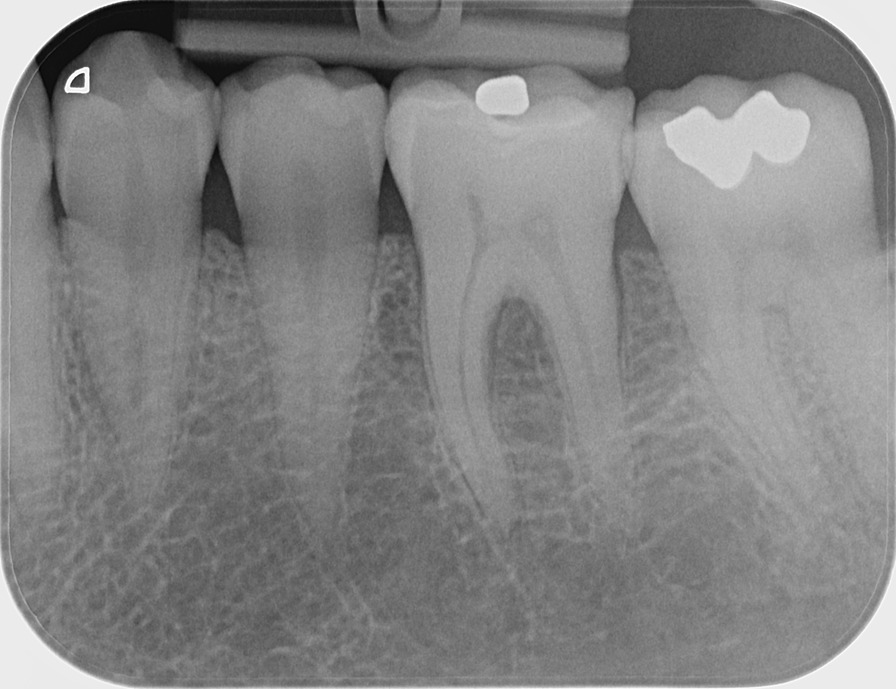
Apical radiograph with a dish-shaped radiolucency in left mandibular first molar region
